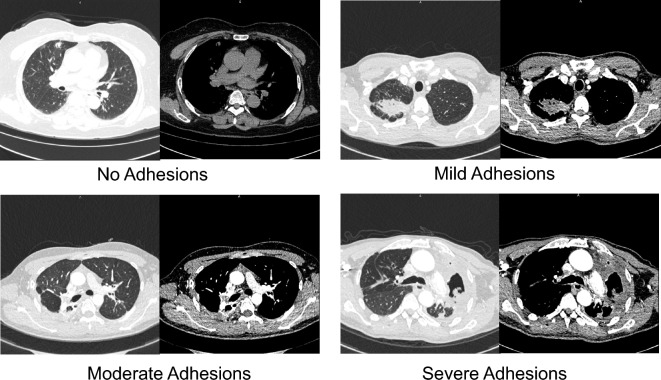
The degree of adhesions between the lung lesion and the pleura on enhanced CT.
